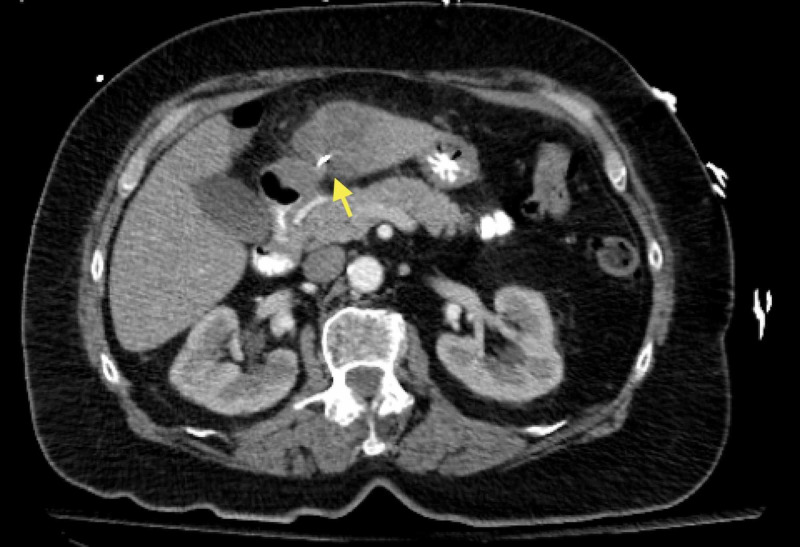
An axial view of the CT abdomen and pelvis showing the metallic foreign body penetrating the liver (yellow arrow)
